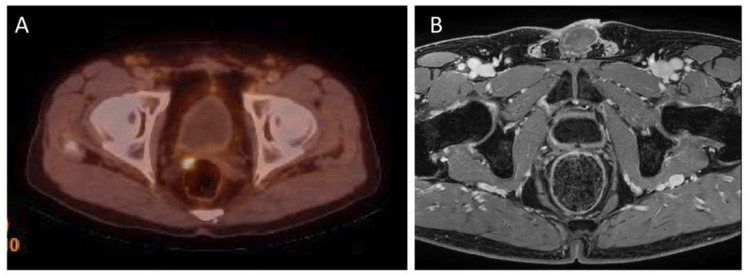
(A)—^18^F-PSMA-1007 PET/CT examination of a patient (74 years old) after radical prostatectomy eight years before, Gleason Score 7(3 + 4), current PSA level 0.29 ng/mL. ^18^F-PSMA-1007 focal uptake in right side tissues adjacent to the prostate bed, in the level under seminal vesicle, pararectaly with SUVmax = 6.9 was detected, confirming local recurrence. (B) this lesion cannot be seen on the corresponding MRI examination (Images from Riga Stradins University Radiology research laboratory archive).
